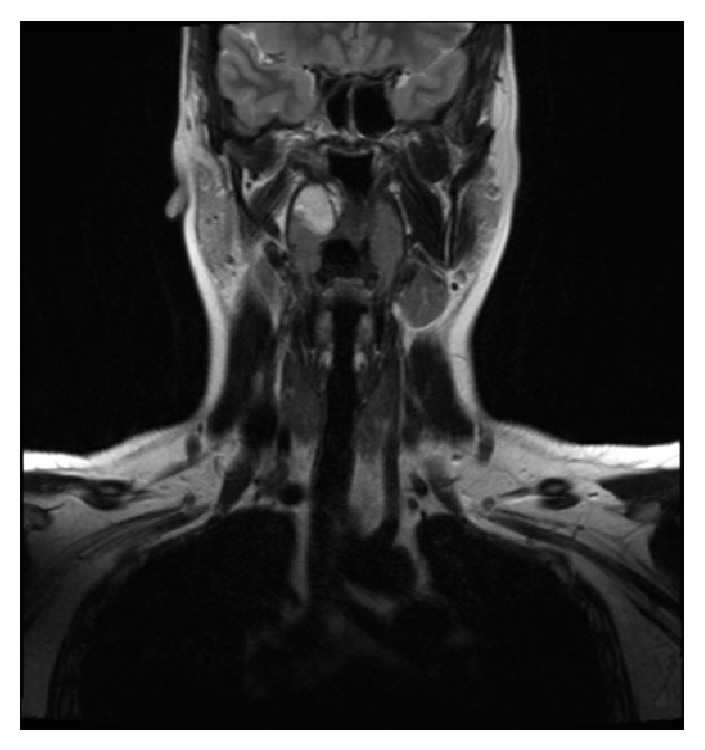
MRI shows a mass in the patient's soft palate.
